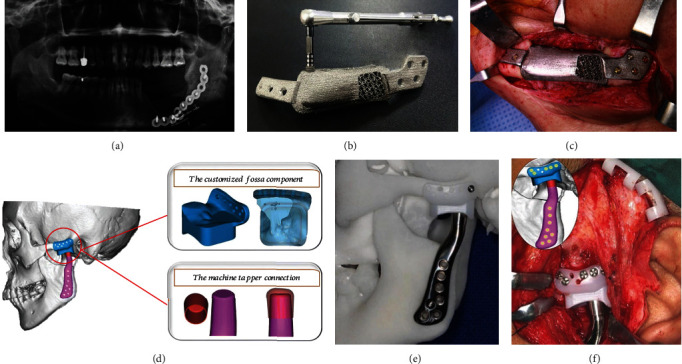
Panoramic radiograph demonstrated huge defect of the left mandible due to osteoradionecrosis (a); a titanium mandibular substitute with premounted dental implant fixtures manufactured by SLM (b) was employed to restore the bony defect (c). Reprinted from [130]. Customized temporomandibular joint (TMJ) prosthesis comprised of the fossa, condylar head, and mandibular handle components (d); the novel TMJ prosthesis can precisely match the Chinese patient's TMJ anatomy (e); the lateral view of a fixed TMJ prosthesis during a surgical procedure (f). Reprinted from [131].
